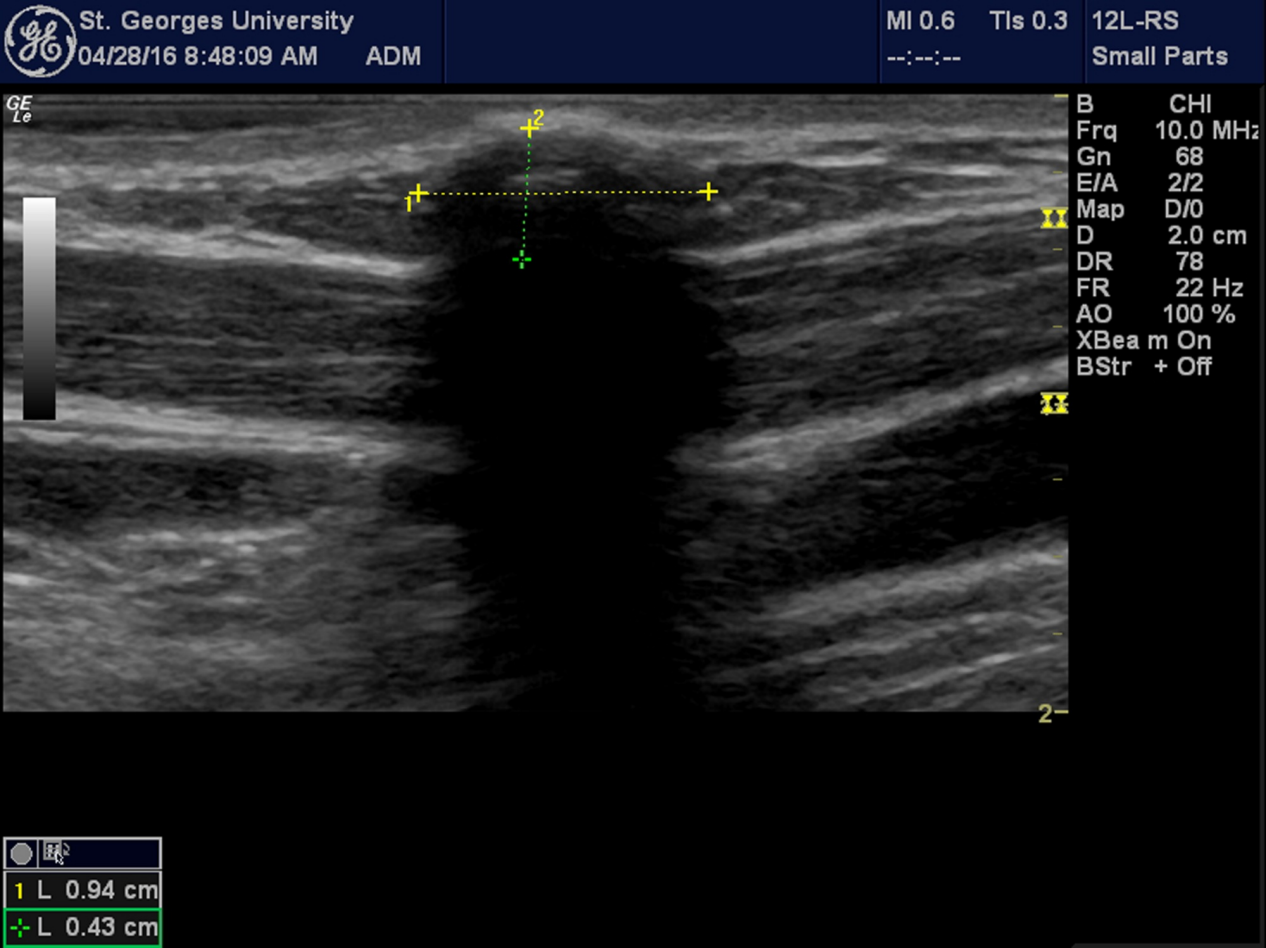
Ultrasound using a linear probe, showing a hypoechoic (likely cartilaginous) mass measuring 0.94 cm x 0.43 cm.
